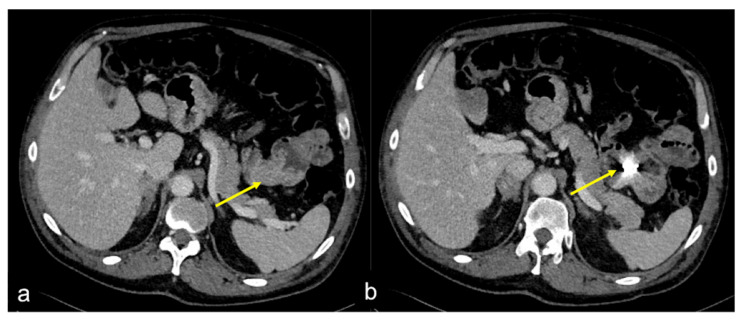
CTA study in venous phase (a) of jejunum adenocarcinoma shows a substenosing solid mass in the lumen of the jejunum (arrow); the successive check after endoscopic haemostasis (b, arrow).
